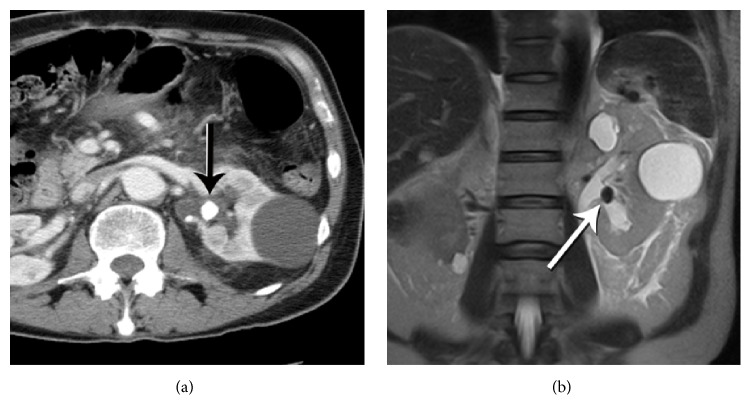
Stone visible on both CT and MR. (a) Enhanced axial CT demonstrates a large stone in the left renal pelvis (arrow). (b) Coronal HASTE MRI image clearly depicts the hypointense stone (arrow) against the backdrop of hyperintense urine.
